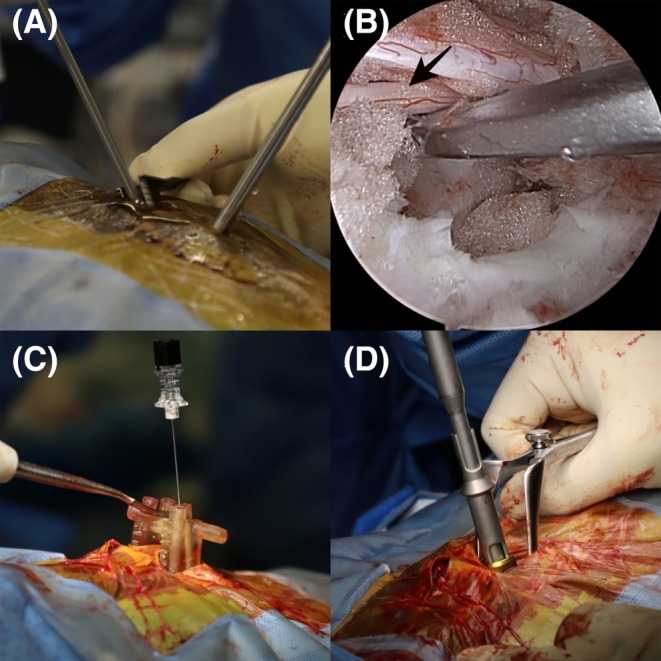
(A) A unilateral biportal endoscopic approach was applied for dorsal laminectomy and discectomy, with creation of a viewing portal and a working portal. (B) Endoscopic view of the lumbosacral junction showing the caudal equina retracted by a probe (black arrow). (C) A three‐dimensional (3D)‐printed patient‐specific drill guide was placed on the sacral region, aligned with the preoperative computed tomography (CT)‐based trajectory. (D) Pedicle screws were inserted into the S1 and S2 vertebrae using a screwdriver and introducer sleeve, following soft tissue retraction with vaginoscope.
